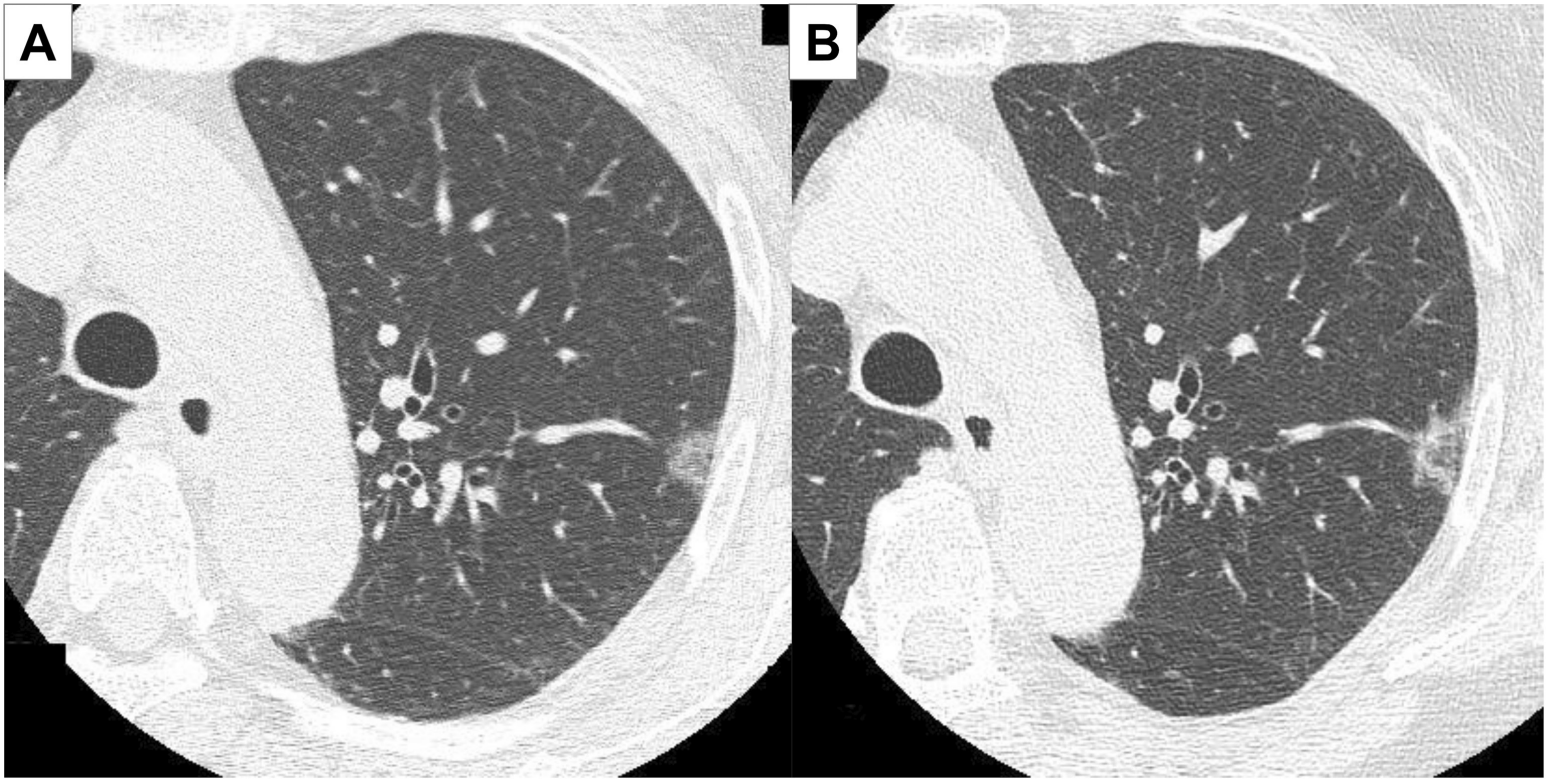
Serial chest computed tomography images showing interval growth of a subpleural ground‐glass nodule with development of the reversed halo sign. (A) Seven years prior to presentation, a subpleural ground‐glass nodule is observed in the left upper lobe. (B) At presentation, the legion is enlarged and exhibits the reversed halo sign.
